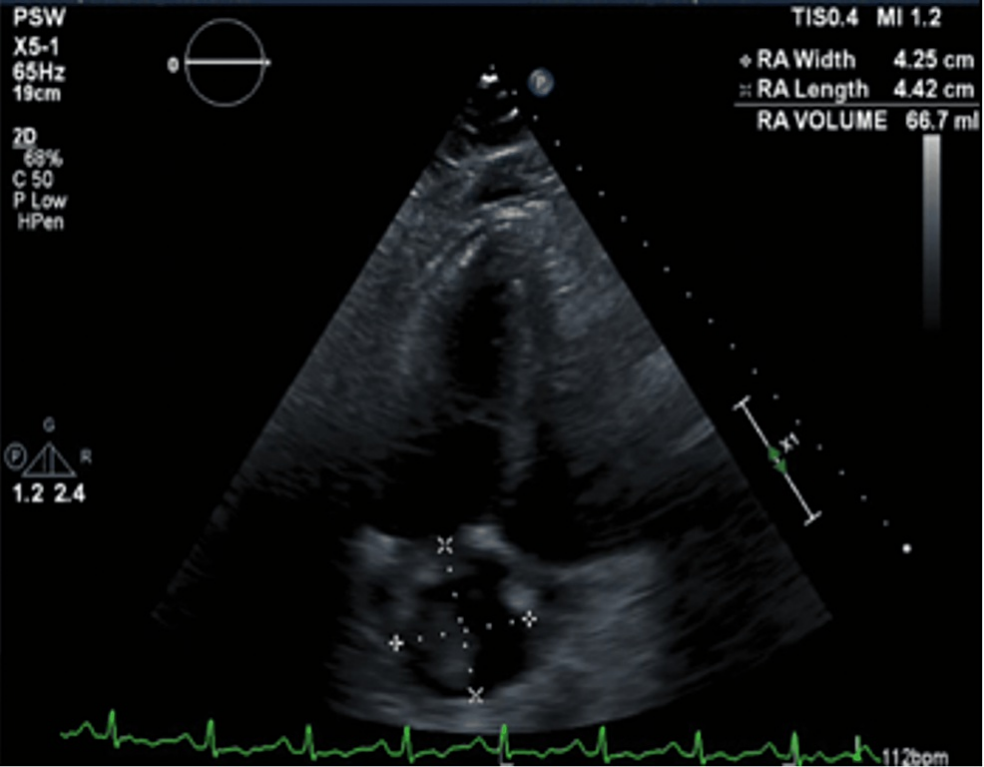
Transthoracic echocardiogram of apical four-chamber view showing right atrial dilation
